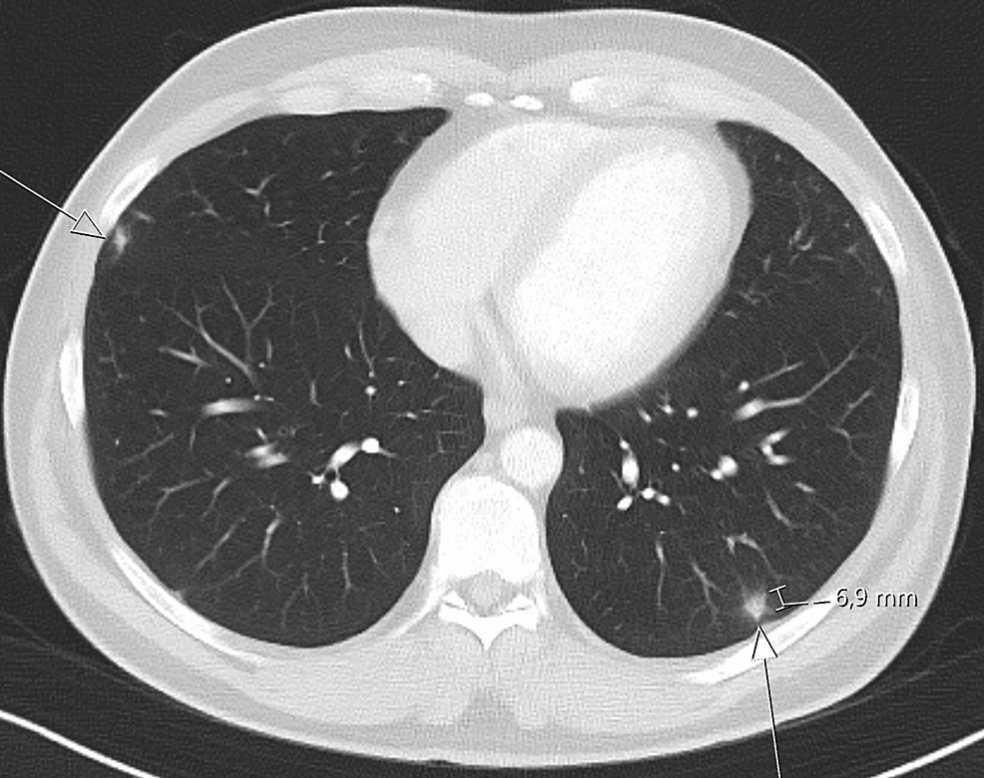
CT scan of the chest at two-month follow up Transversal image showing significant labeled regression of the lesions with the largest lesion up to 6.9 mm in diameter
